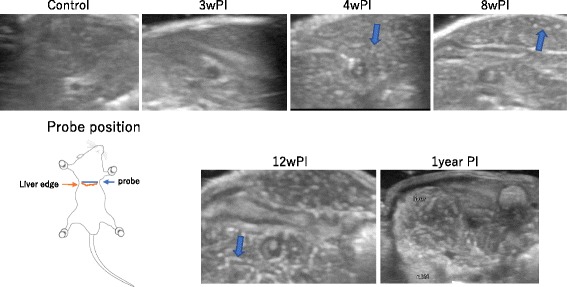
Change of the liver parenchyma in ultrasonography. At 3 weeks PI, there was no marked change in the liver parenchyma and spots appeared from 4 weeks PI (arrow). After that, the spots were enlarged, and at 8 weeks PI, a thin linear change was also detected (arrow), which became more prominent at 12 weeks PI (arrow). A thick linear change was conspicuous at 1 year PI
